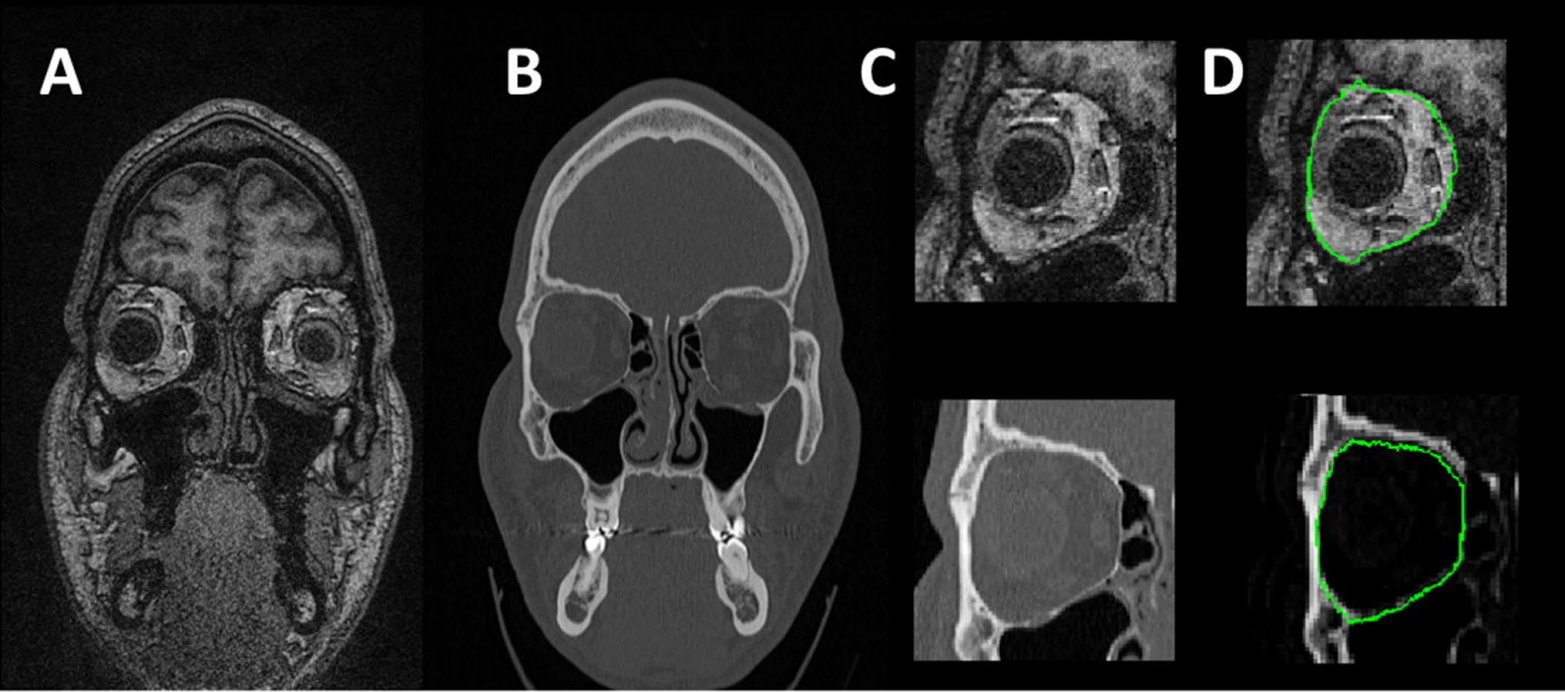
An example of a coronal plane image from the dataset for both T1-weighted 3 T MRI (A) and CT (B). Images belong to the same subject and represent approximately the same cross-section. A subplot of the region of interest (right orbit) for both imaging modalities is shown without (C upper panel MRI, lower panel CT) and with (D upper panel MRI, lower panel CT) the boundaries of the intraorbital bony contours in green.
